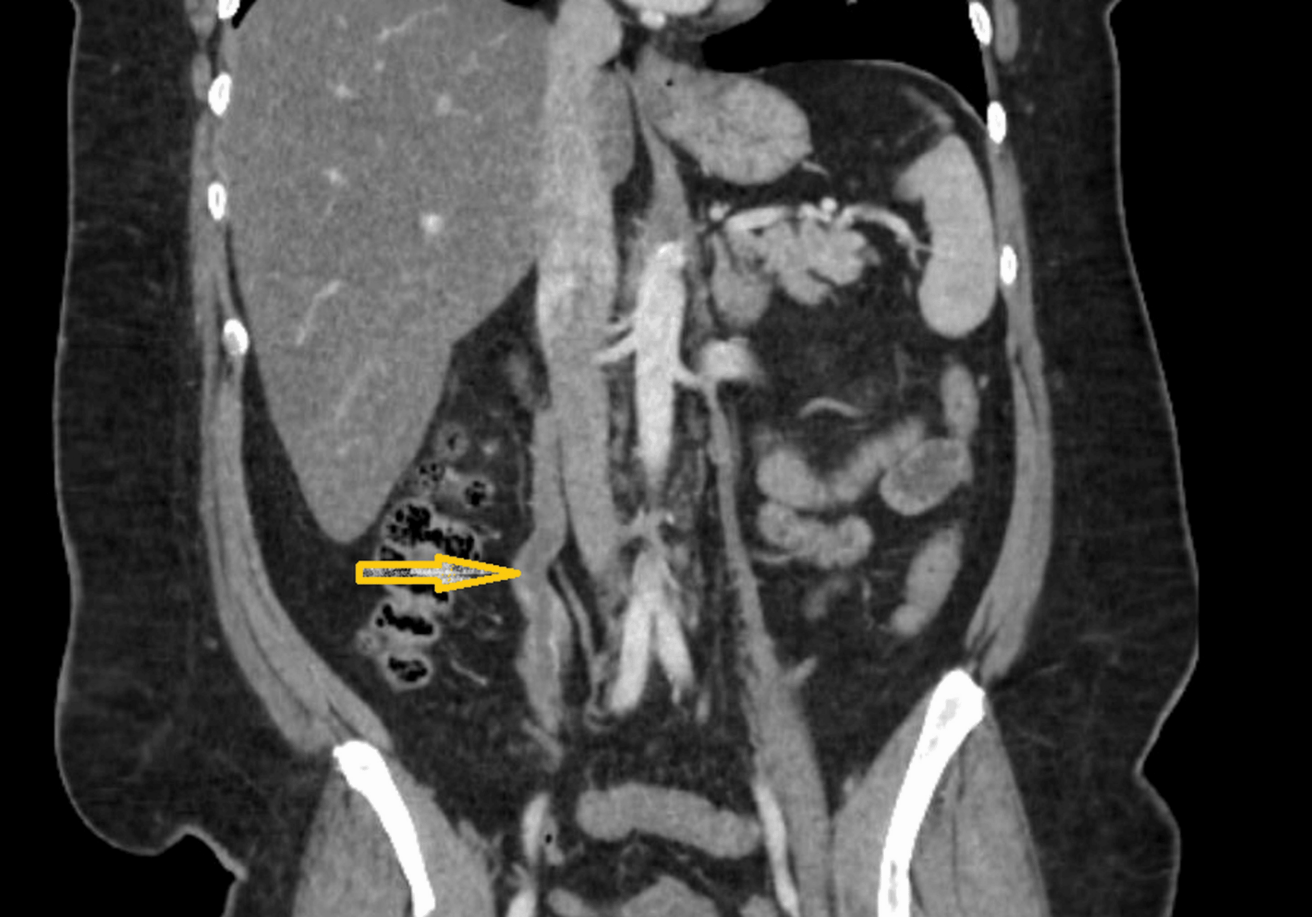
Contrast-enhanced computed tomography (CT) of the abdomen showing acute right gonadal vein thrombosis. Coronal contrast-enhanced CT image demonstrating a filling defect within the right gonadal vein (yellow arrow), consistent with acute thrombosis.
